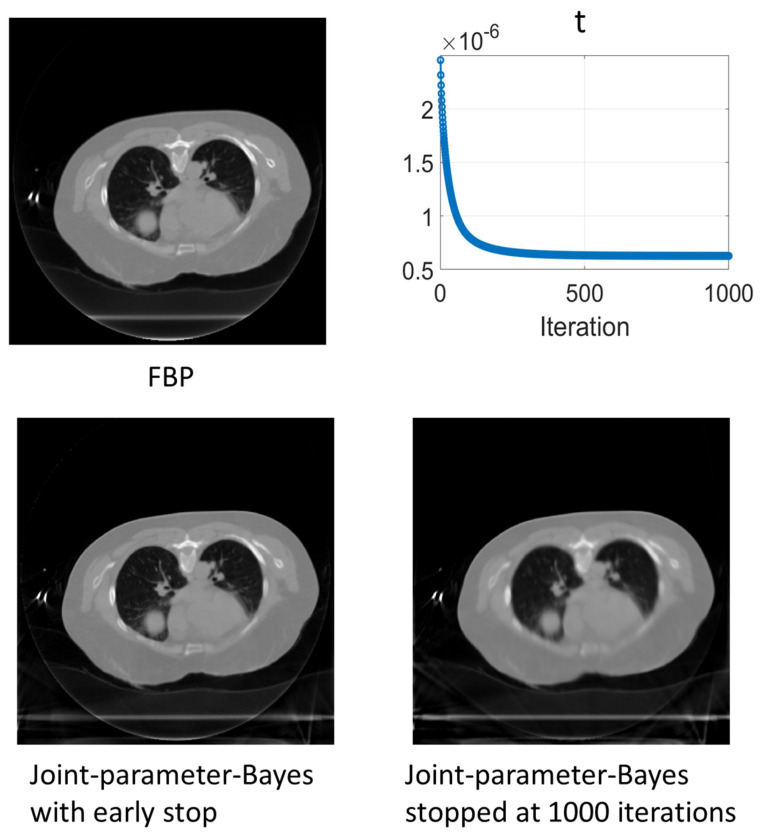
Comparison of reconstructed images between the conventional MAP and joint-parameter-Bayes. The CT image display window is [0, 0.035] mm^−1^.
